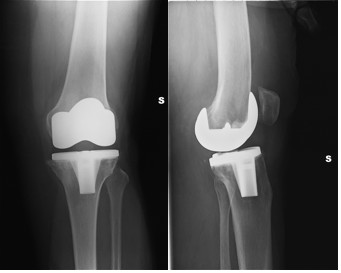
Preoperative X-rays. The hyperextension of the tibia in relation to the posterior subluxation of the femur can be seen.
